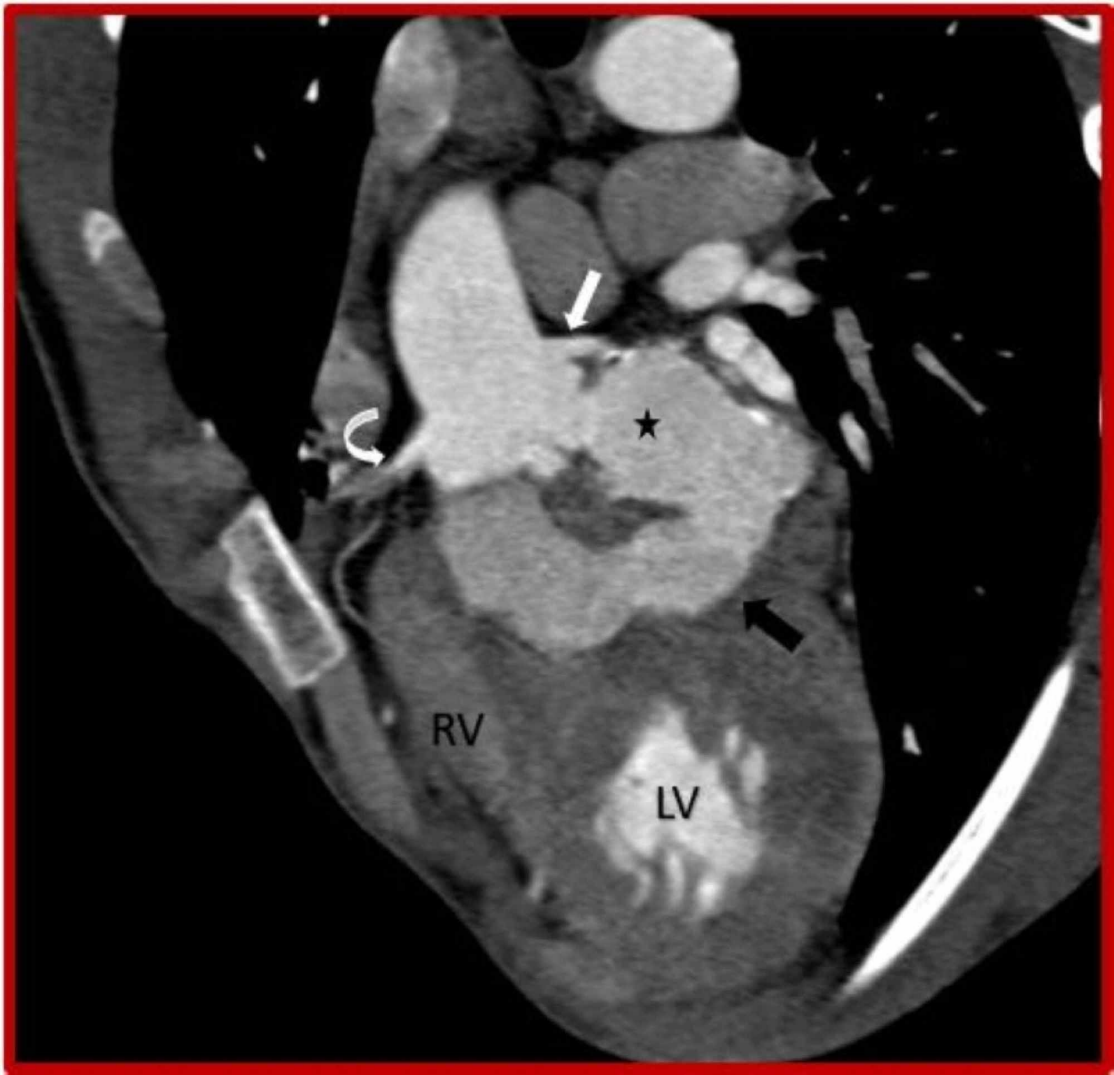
Cardiac CT (coronal oblique view) demonstrates ruptured left sinus of Valsalva aneurysm (asterisk) dissecting into the interventricular septum (black arrow) from the basal to mid septal region. CT: Computed tomography; Curved arrow: Right coronary artery; Straight white arrow: Left coronary artery; RV: Right ventricle; LV: Left ventricle.
